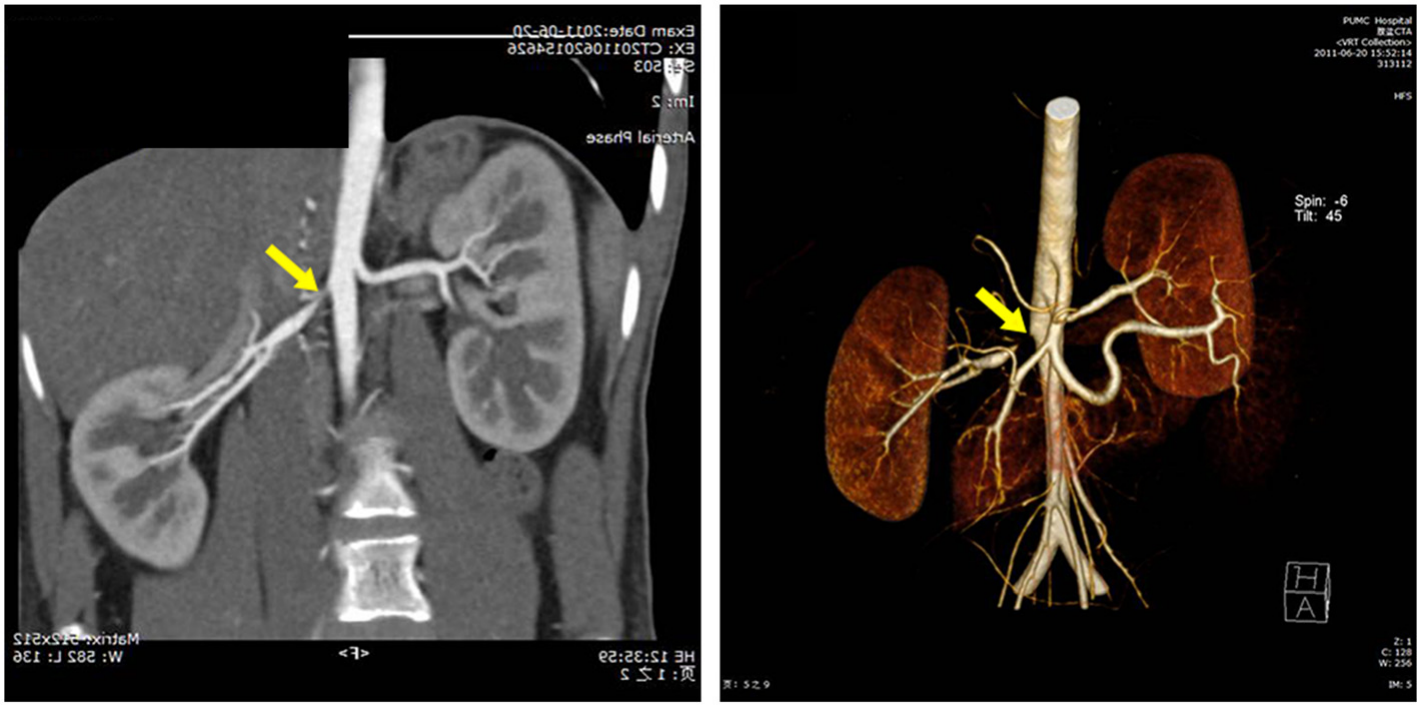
Computed tomography angiography of the renal vessels. The initial segment of the right artery was almost occluded, but there was a collateral circulation from the subphrenic artery. Blood flow was 36.7 ml/minute in the right renal, and 57.1 ml/minute in the left.
